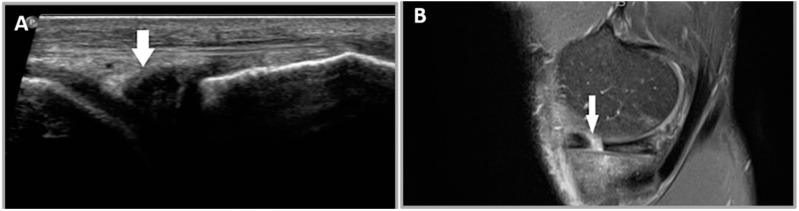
A 28-year-old female patient who presented with a history of left knee injury and medial sided knee pain. (A) A meniscal cleft is seen as a hypoechoic defect in the body of the medial meniscus (white arrow). (B) A sagittal proton density MRI image demonstrates a hyperintense defect in the body of the medial meniscus in keeping with a radial tear (white arrow).
